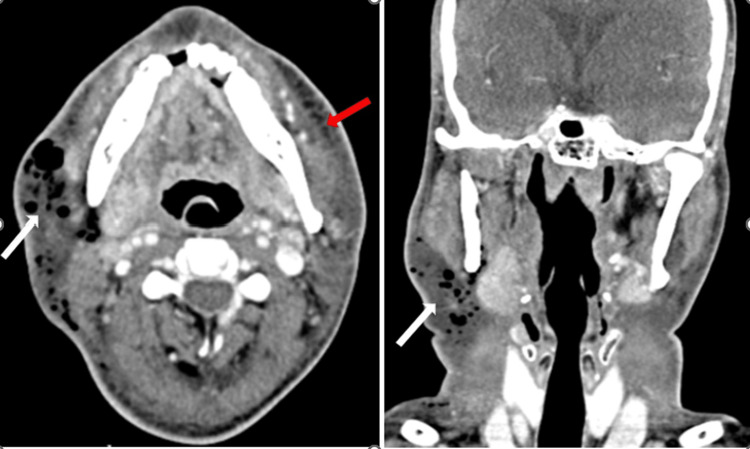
(Case 1) Contrast-enhanced CT of the neck (axial and coronal) shows an extensive ill-defined fluid collection (white arrows) containing multiple air pockets involving the subcutaneous plane of the neck bilaterally and extending into the right submandibular space. Note the absence of a peripheral rim around the fluid collection. Significant soft tissue edema and stranding is also seen (red arrow).
